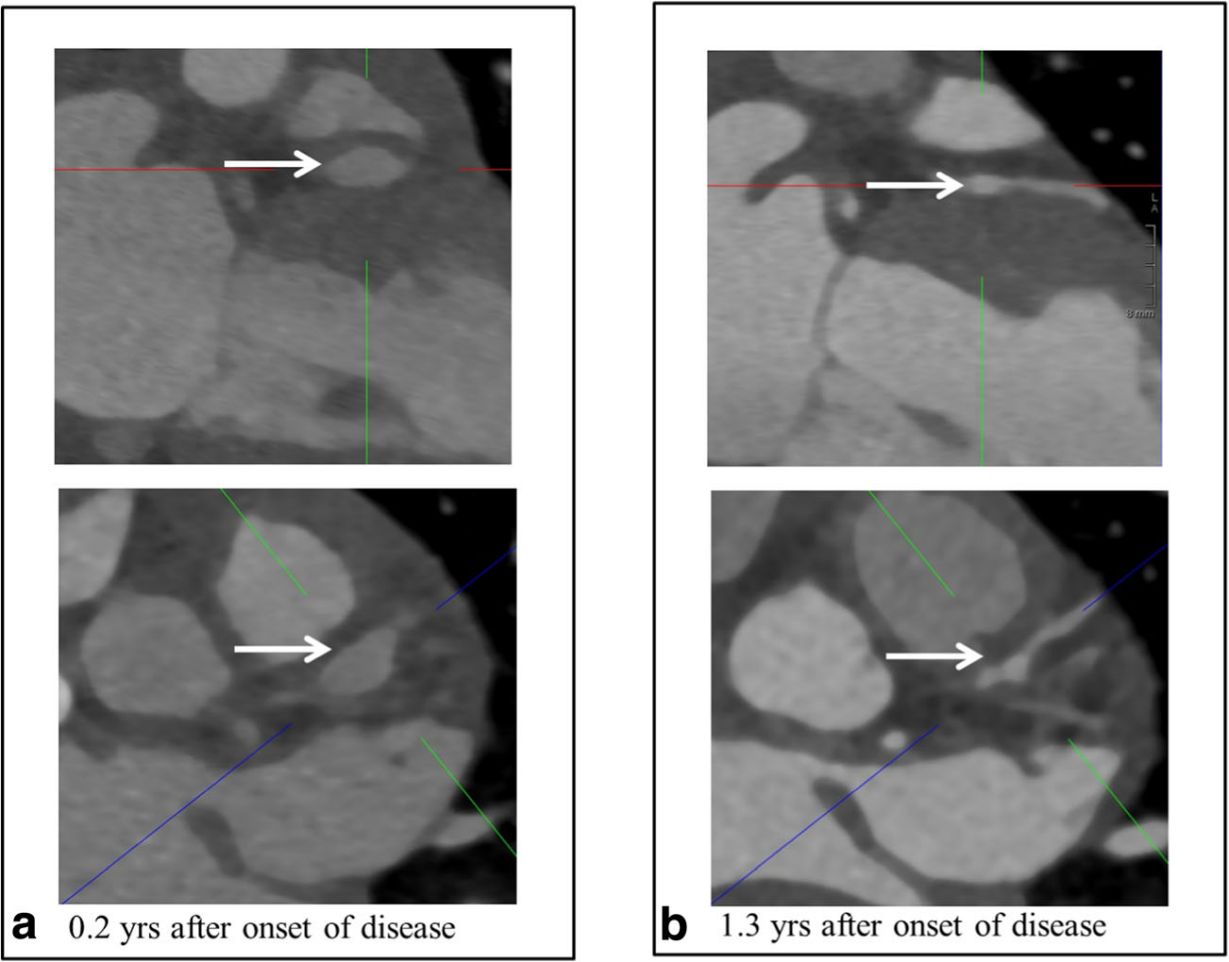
Remodeling of the LAD (in a single patient) performed with the third-generation dual-source CT scanner. a Significant aneurysms in the LAD (5.3-mm diameter, *Z* score 16.28). b Remodeling of the LAD in the dynamic phase (2.8-mm diameter, *Z* score 4.23)
